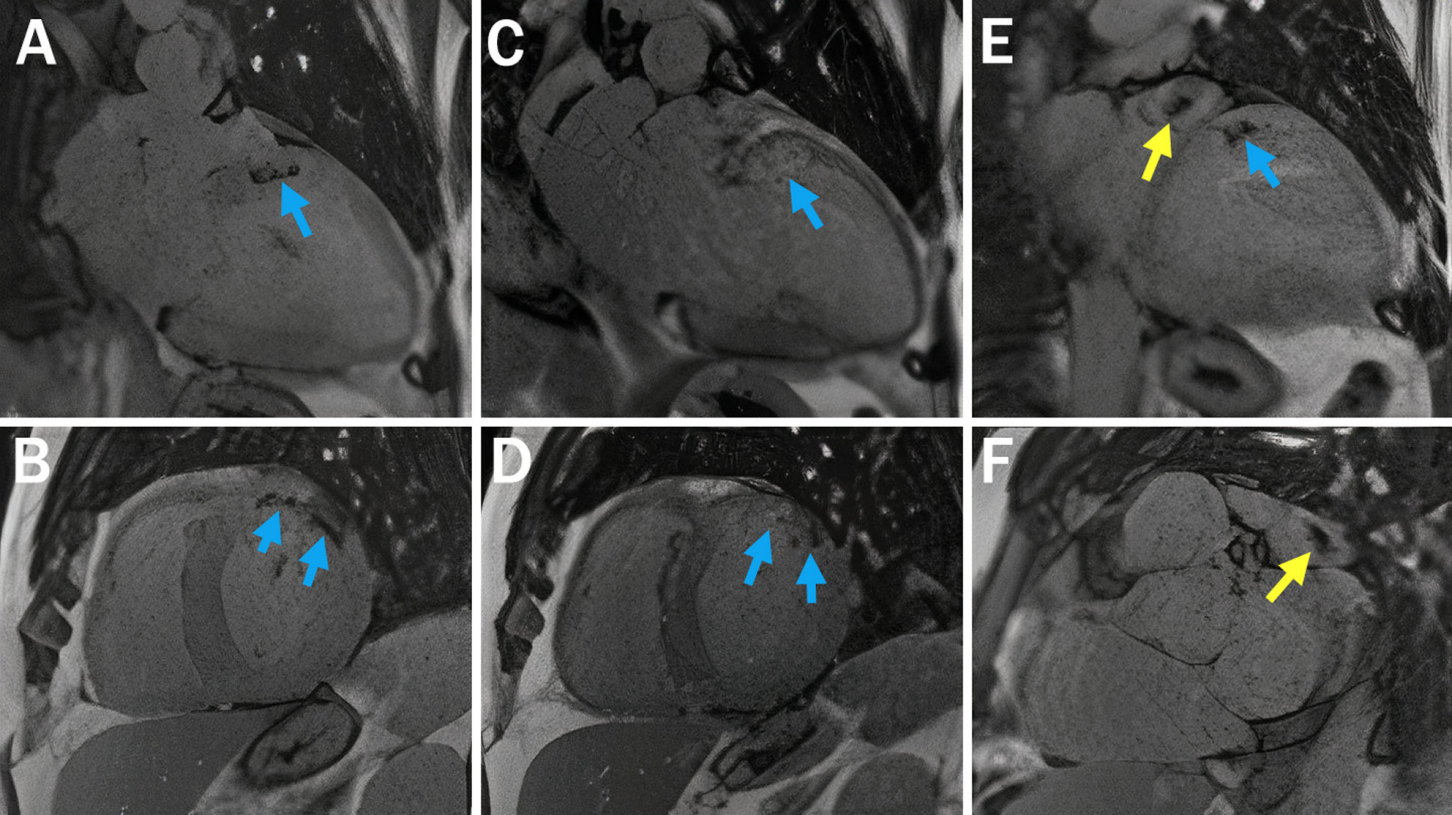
Cardiovascular magnetic resonance imaging with inversion recovery sequence using long inversion time showed area of no contrast uptake at the basal anterior and anterolateral wall (A, B, E, blue arrows) that improved on the similar sequence repeated 30 minutes later (C, D, arrows) consistent with microvascular obstruction. (E, F) Left atrial appendage thrombus (yellow arrow) was also discovered on this sequence. Image enlarged using letsenhance.io.
